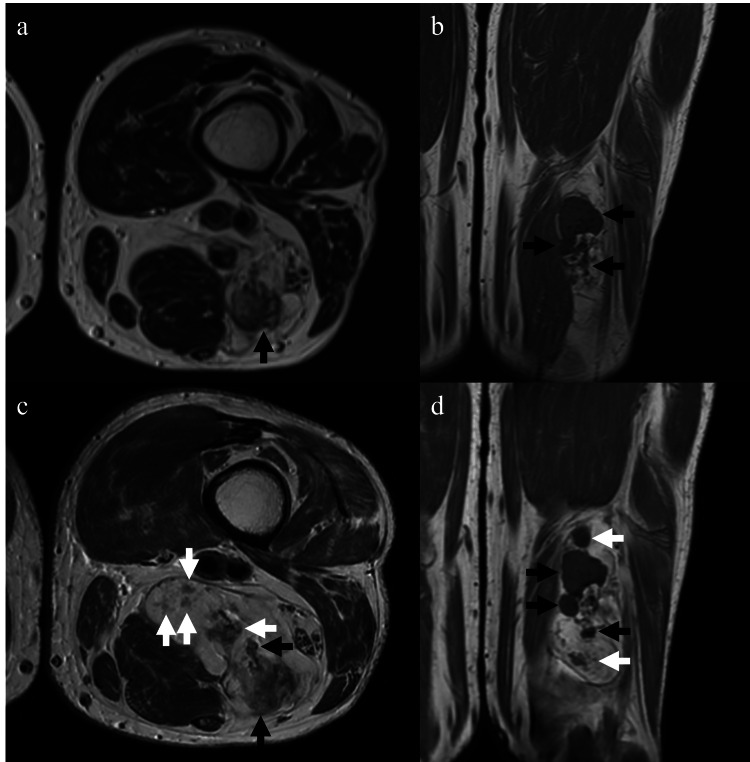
DDLPS with low-grade osteosarcomatous differentiation of the left femur in an 80-year-old male presenting no symptoms Initial MRI (a, b) includes high-intensity areas corresponding with fat and low-intensity areas corresponding with calcification (black arrows) on axial T2-weighted MRI (a) and coronal T1-weighted MRI (b). Follow-up MRI (c, d) reveals noticeable enlargement (black arrows) and new development (white arrows) of the calcified areas, as well as fatty areas. MRI: magnetic resonance imaging, DDLPS: dedifferentiated liposarcoma
